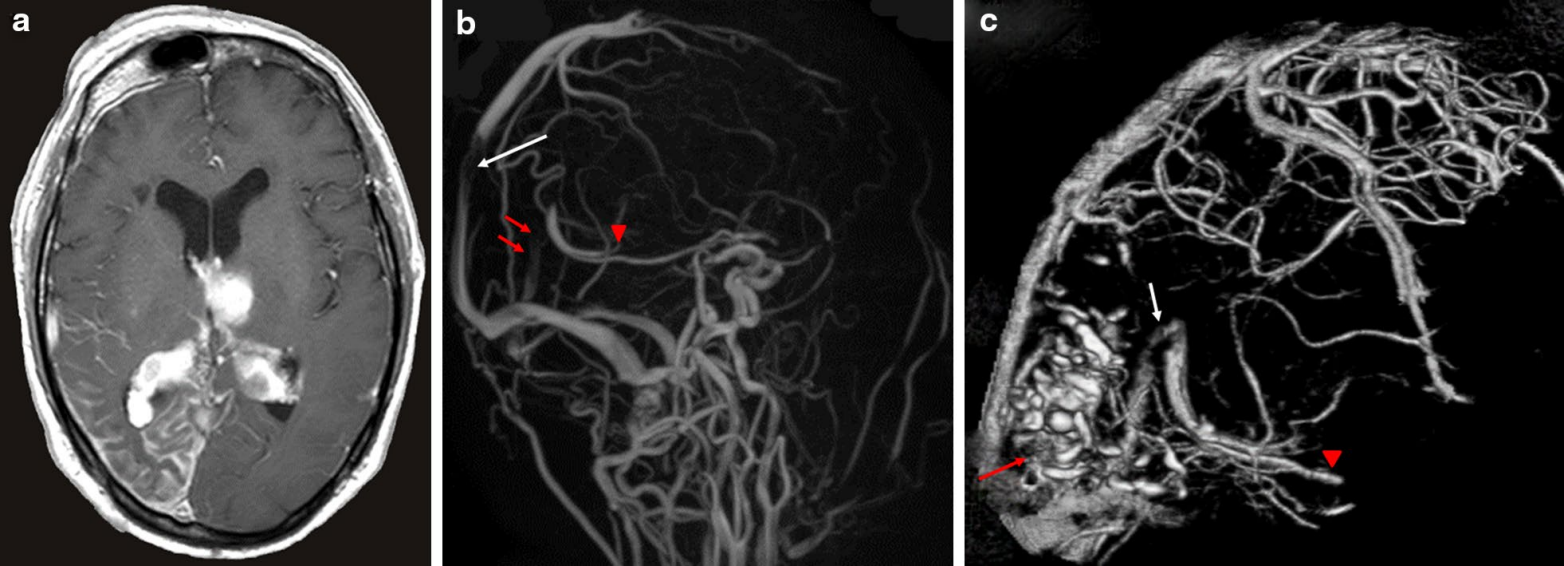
a Gadolinium-enhanced MRI showing left thalamic hemorrhage, leptomeningeal enhancement in the right temporo-occipital lobe, and choroid plexus thickening and enhancement on both sides. b Magnetic resonance venography (right to left view) showing steno-occlusion of the superior sagittal sinus (*white arrow*), straight sinus (*red arrows*), and left internal cerebral vein (*red arrow head*). c Three-dimensional CT angiography 2 weeks after the onset (right to left view) showing the patency of the straight sinus (*white arrow*) and left internal cerebral vein (*red arrow head*) and calcification in the right occipital lobe (*red arrow*)
